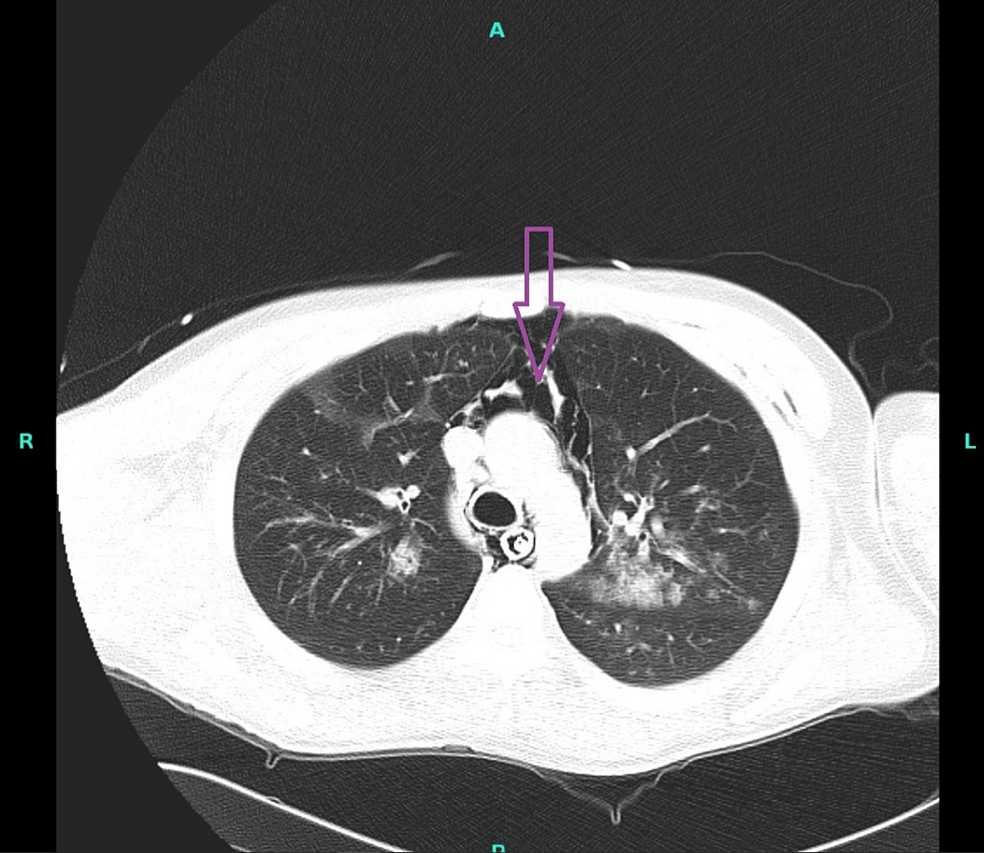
CT chest without intravenous contrast showing pneumomediastinum (purple arrow)
